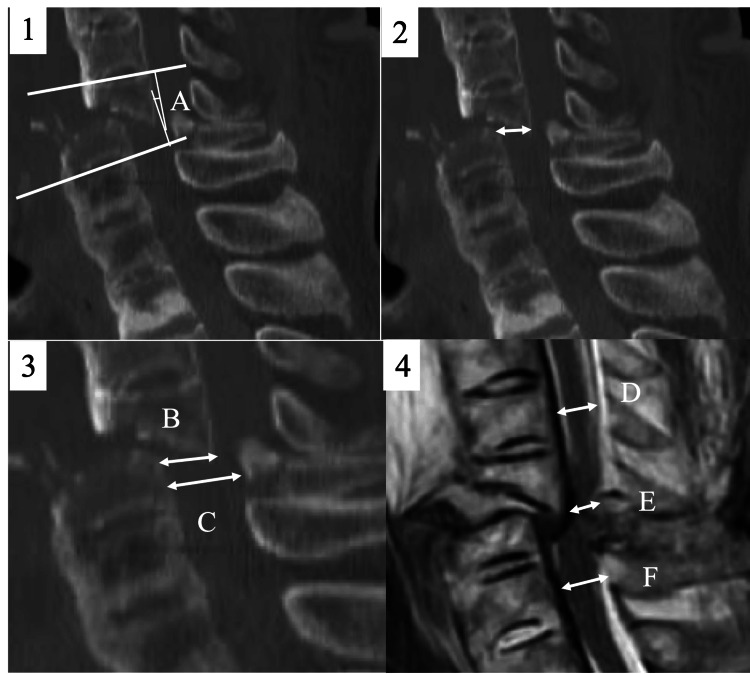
Radiographic parameters 1: Fracture angle: Fracture angle ("A" in figure) was defined as the angle between the superior and inferior margins of the fractured vertebra in the CT sagittal image. 2: Fracture displacement distance (mm): The displacement distance was measured in the slice with the greatest vertebral separation due to fracture in the CT sagittal images. 3: Spinal canal stenosis rate on sagittal CT images: stenosis rate (%) = B/C×100%. 4: Dural stenosis rate on sagittal MR images: dural stenosis rate (%) = ((D+F)/2-E) /(D+F)/2×100%.
